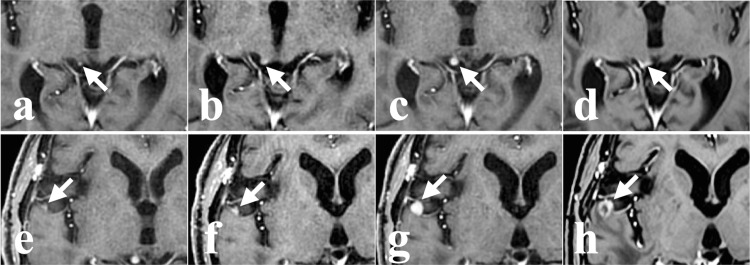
Radiographic evolution of presumed CSF dissemination on axial enhanced MRI (a-d) Right midbrain lesion and (e-h) right temporal lobe lesion at various time points post-surgery. White arrows indicate the tumors. (a) At 28 months, a small new lesion was retrospectively identified. (b) At 35 months, before SRT for the enlarging lesion. (c) At 37 months, the lesion showed a roundish expansion. (d) At 63 months, the lesion had collapsed. (e) At 37 months, a small new lesion was retrospectively identified. (f) At 40 months, showing lesion growth. (g) At 45 months, before SRT. (h) At 65 months, MRI showing central necrosis within the lesion. CSF; cerebrospinal fluid, MRI; magnetic resonance imaging, SRT; stereotactic radiotherapy
